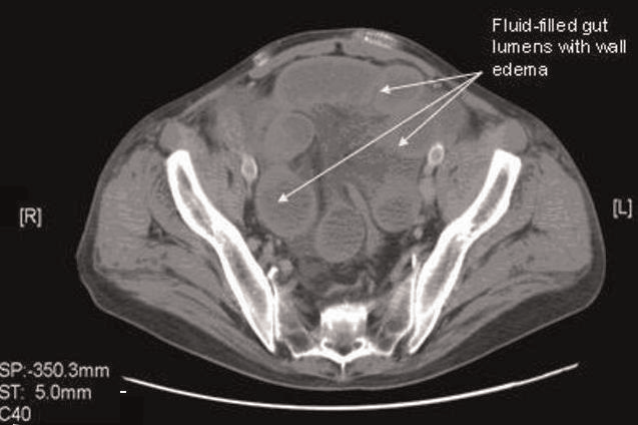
CT abdomen with and without contrast showing fluid-filled gut lumens with wall edema.
